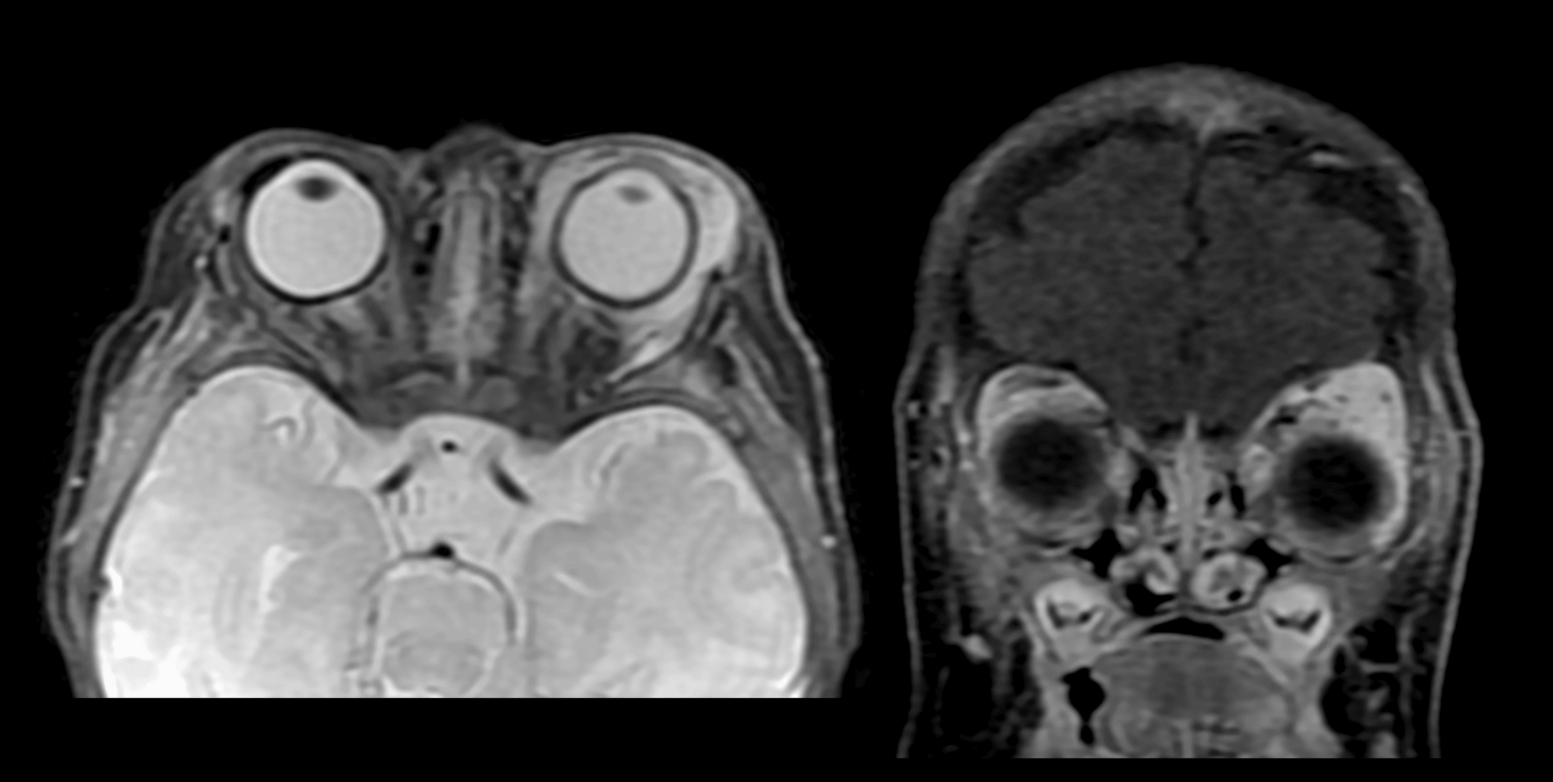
Facial MRI showed a left orbital hemangioma with pre- and post-septal extension. The lesion caused significant eyelid swelling, leading to restricted mobility and partial obstruction of vision.
